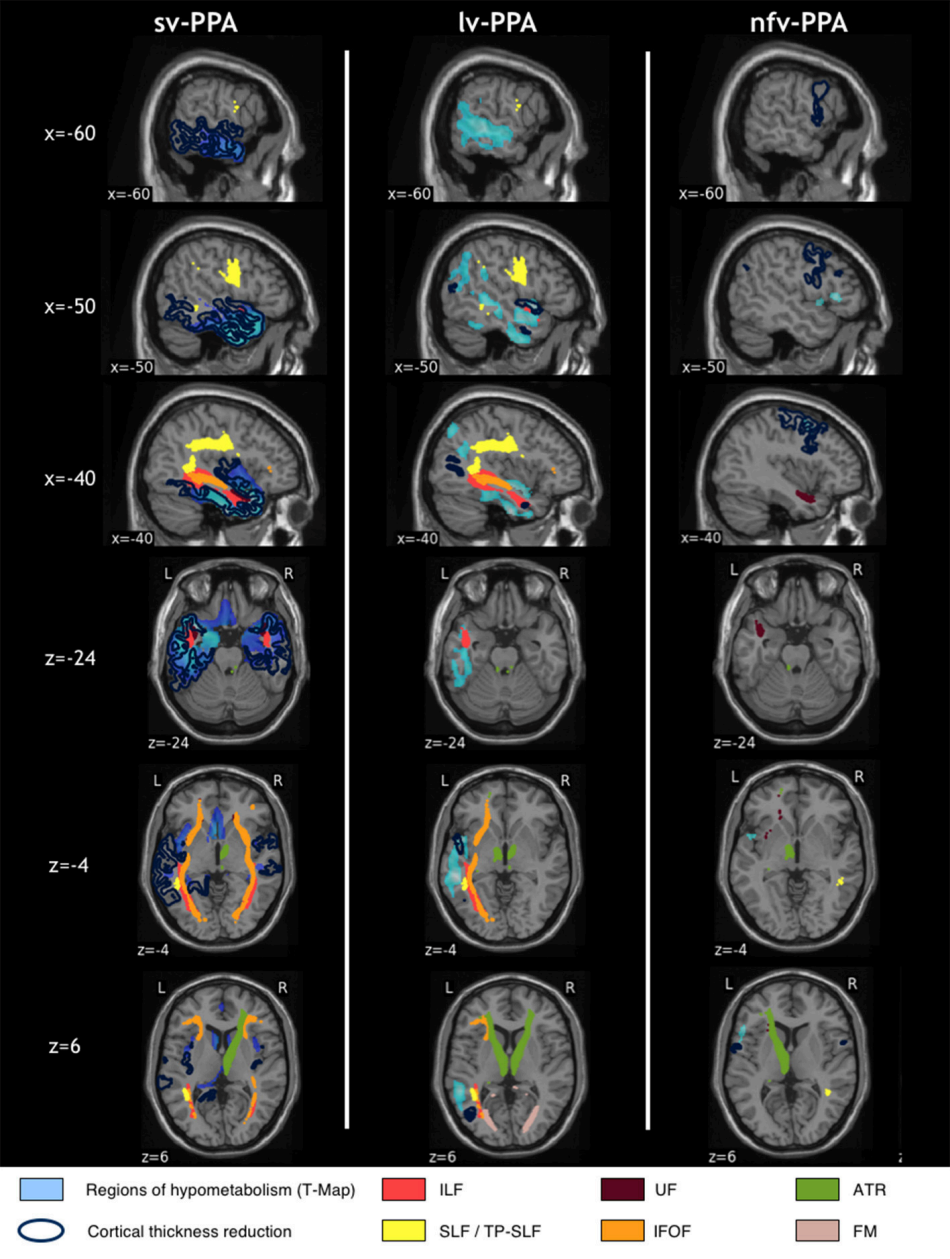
Side-by-side visualization of multimodal alterations in sv-PPA, lv-PPA and nfv-PPA, compared to healthy controls. Significantly altered areas correspond to a corrected *p*-value of 0.05 for all modalities. The correction for multiple comparisons used FWE at the vertex-level for T1, FWE at peak-level for PET and Bonferroni for DWI.
